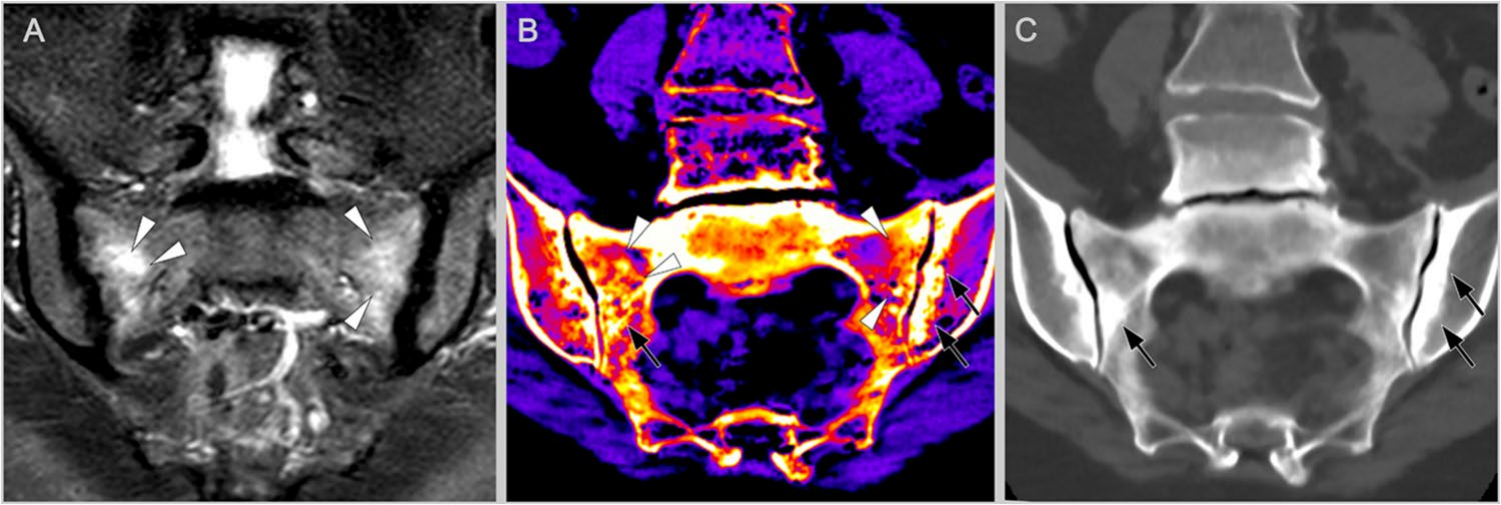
DECT of the sacroiliac joints in Osteitis condensans ilii. MRI-STIR (A), DECT virtual non-calcium maps (B) and conventional CT reconstructions (C) of a 59-year-old female patient with bilateral bone marrow oedema (arrowheads) and sclerosis (arrows) in Osteitis condensans ilii. In the bone marrow reconstructions (B), normal bone marrow is black to blue and oedema red to yellow. Especially on the left sacrum, it is hard to distinguish oedema and sclerosis. Therefore, a careful comparison of standard CT reconstructions and DECT maps is necessary, and results have to be interpreted with caution
